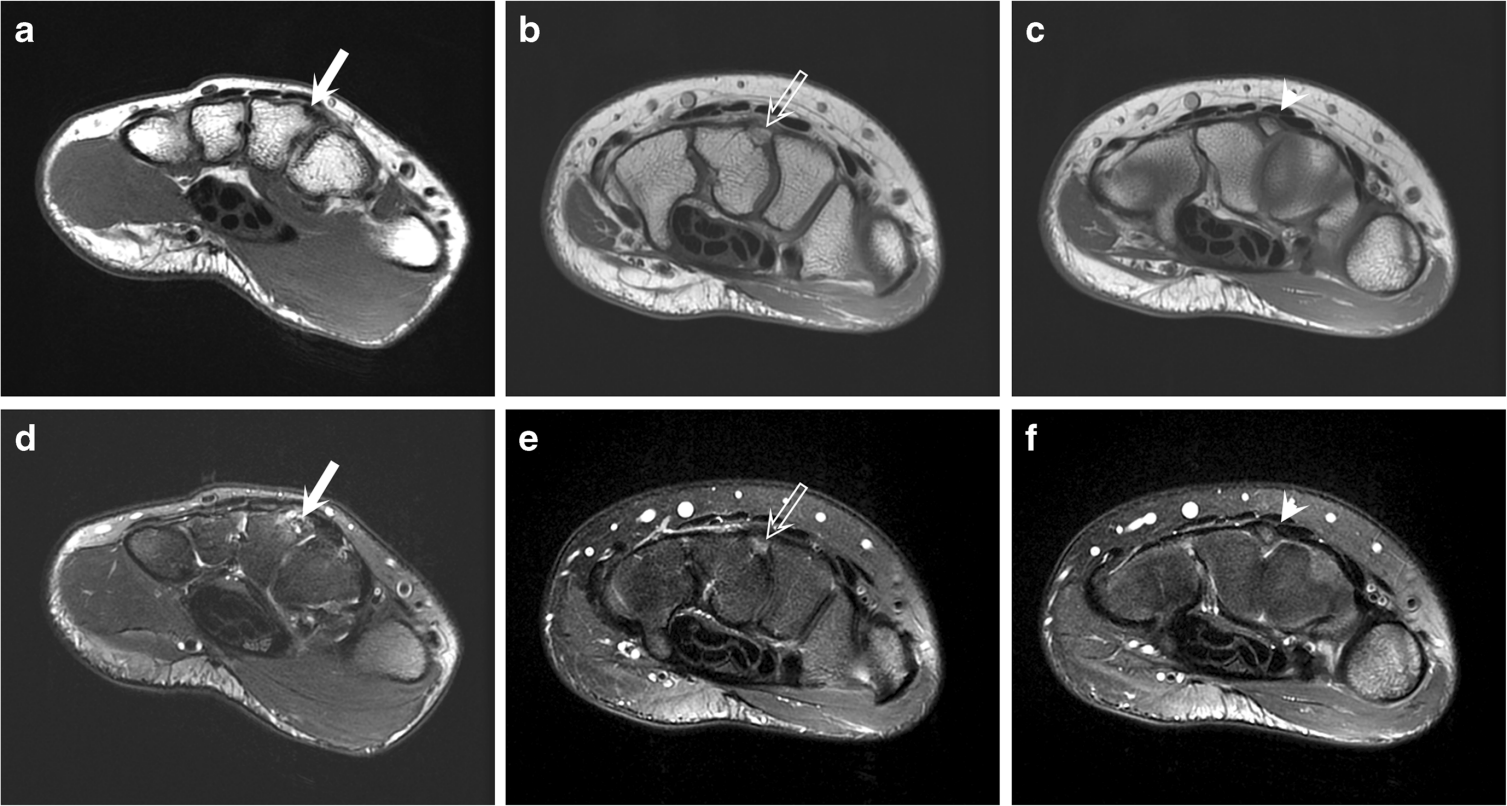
a–c T1-weighted and d–f T2-weighted fat-saturated axial MR images of the wrist depicting a fused carpal boss (a, d), a partial coalition (b, e), and an os styloideum (c, f). A 46-year-old man with an osseous prominence, i.e., fused carpal boss (*white arrow*) on the base of the 3rd metacarpal with degenerative changes (a, d). A 21-year-old woman showing a partial coalition of the os styloideum with mild bone marrow edema (*open arrow*; b, e). A 24-year-old man demonstrating a characteristic unfused os styloideum (*arrowhead*; c, f)
